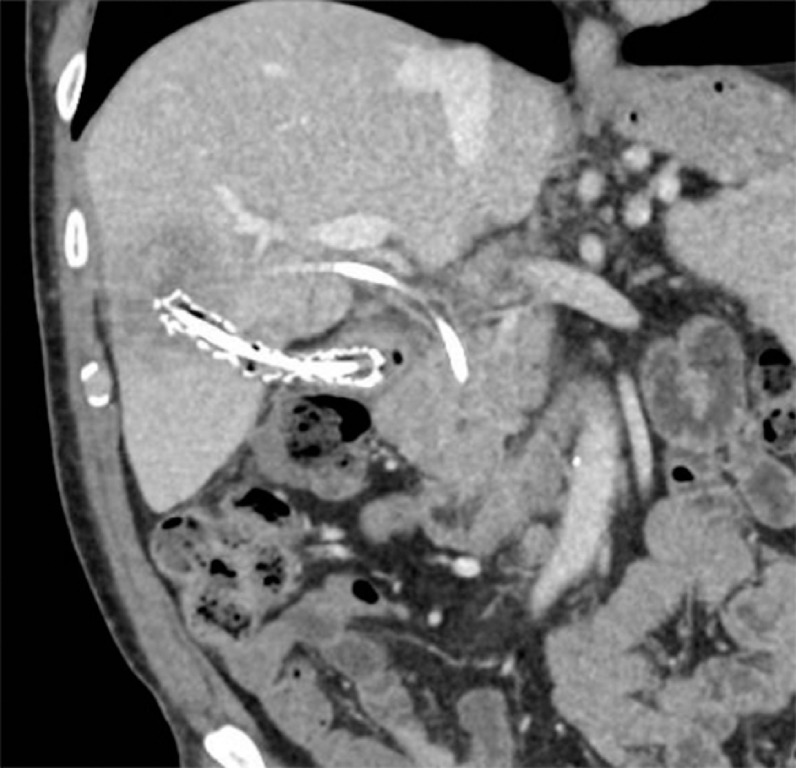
Follow-up computed tomography scan 1 month after the procedure showing abscess resolution and reduction in the biliary dilatation.
